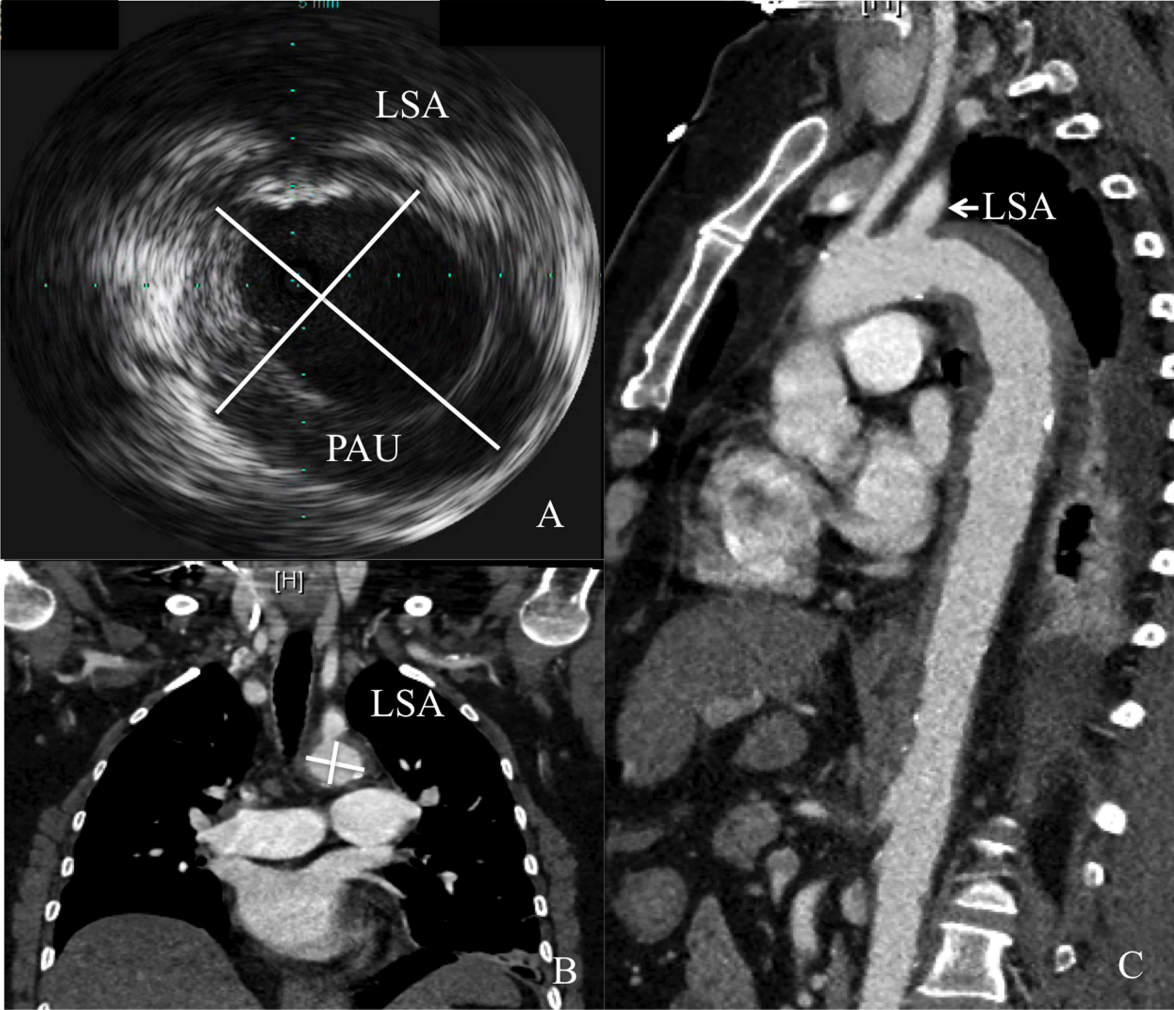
Example for measurement of the aortic diameter at level of the left subclavian artery (LSA) with Type B aortic dissention (+). CT (A) and IVUS (B) measurements at the level of the LSA (*). Penetraiting aortic ulcer (PAU) is shown in IVUS.
